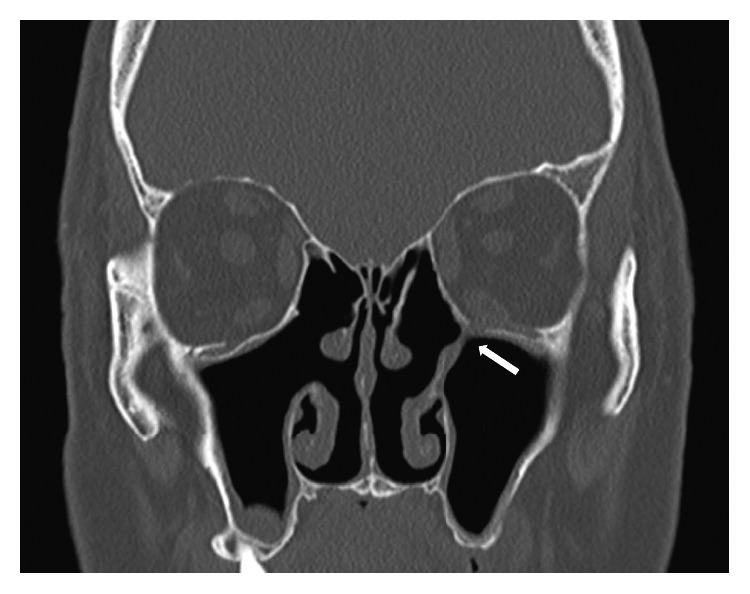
CT scan of the sinuses without contrast, frontal view. 5 mm defect in the posterior medial orbital floor with inferior displacement of the inferior rectus muscle into the defect. Soft tissue band was present from the defect to the remnant of the uncinate process (arrow).
